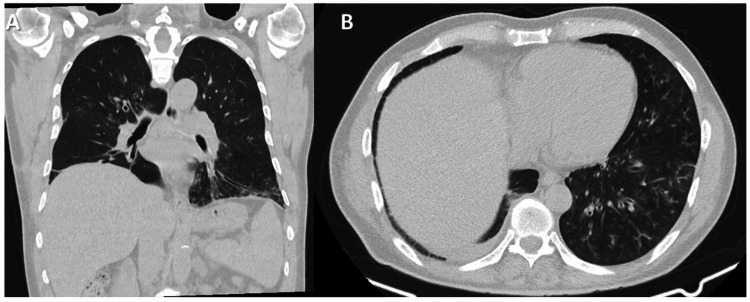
(A and B) Coronal and axial chest CT show centrilobular ground-glass nodules, tree in buds, and thickened airways mainly at the left lower lung lobes.
